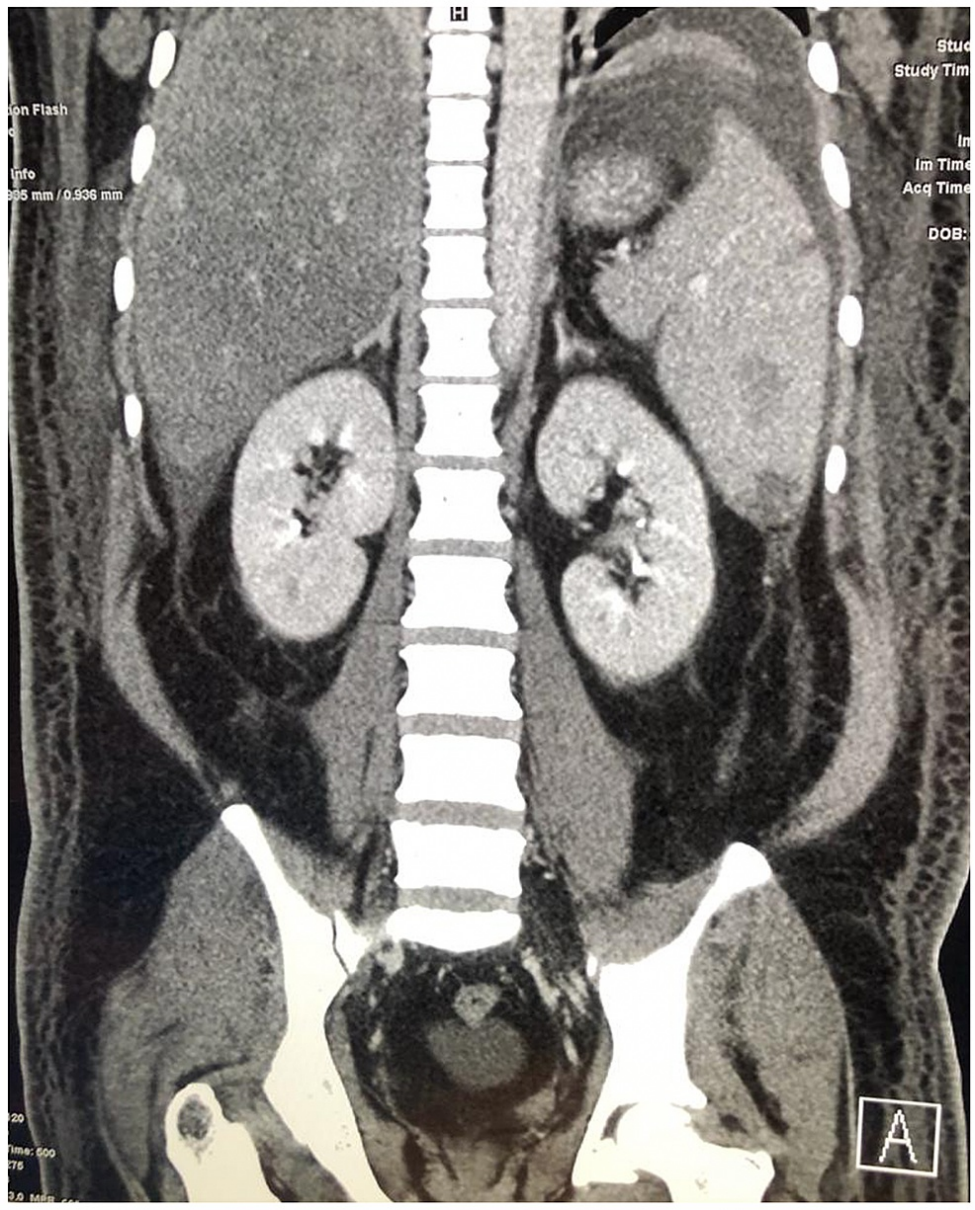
Abdominal CT shows multiple hypodensities across the spleen.
